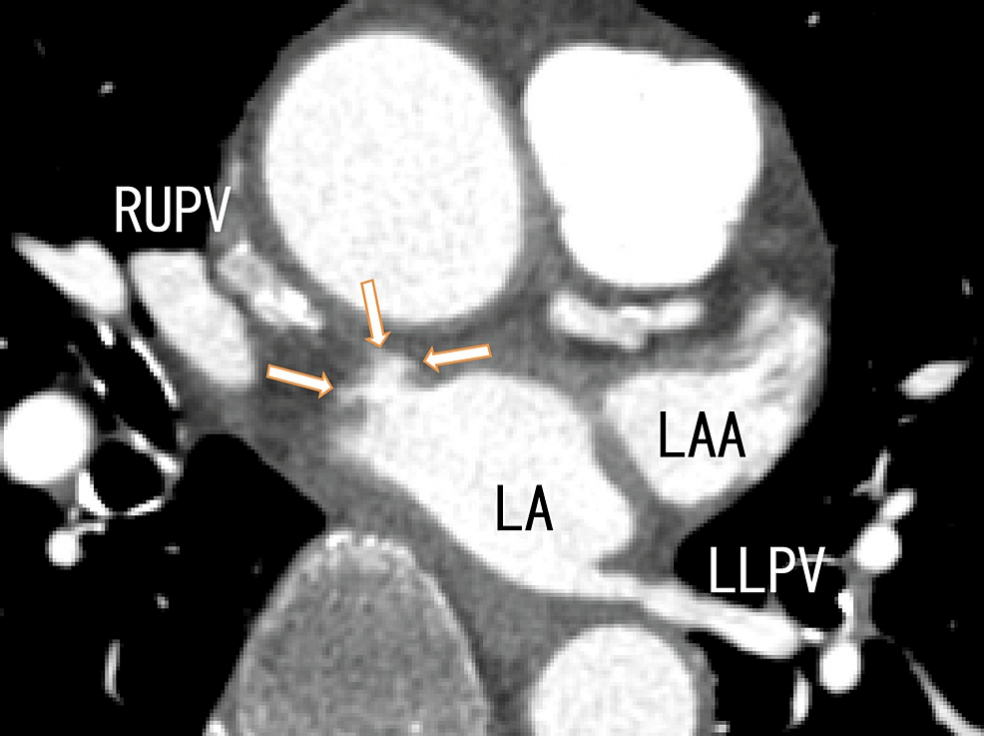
Axial images from an 80-MDCT scan obtained after one month of heparin and warfarin treatment The location was similar to that of the 80-MDCT scan images in Figure 4. There was a diverticulum on the anterior wall of the LA (arrows). There was a little dark area in the LA, which was situated at the root of the diverticulum. The dark areas of the RUPV and LA became clearer, indicating that the thrombi had resolved. LA, left atrium; LAA, left atrial appendage; LLPV, left lower pulmonary vein; RUPV, right upper pulmonary vein; MDCT, multidetector computed tomography
